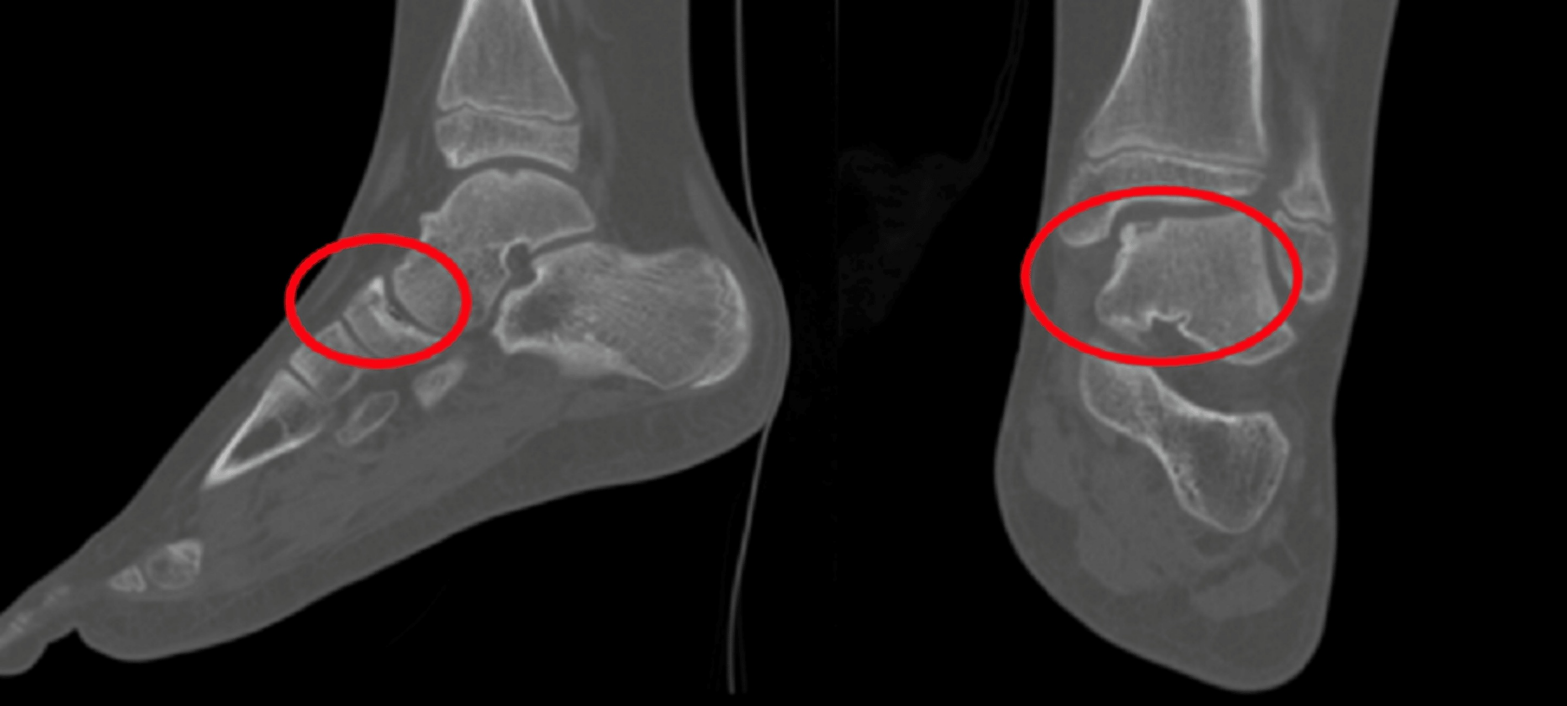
Left ankle computed tomography imaging showing osteochondral defects of the talar dome and navicular bone (Stage I-II) (circles).
